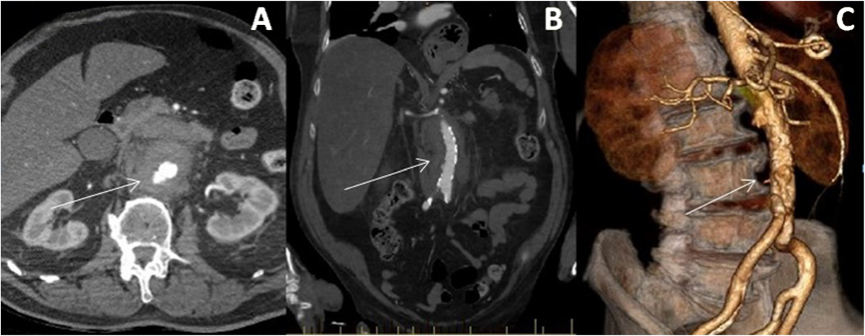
Imaging of the abdominal aorta prior to surgery. A. CT showing inflammatory tissue surrounding the leaking abdominal aorta (transverse plane). B. Coronal plane showing the abdominal aorta. C. Volume-rendered reconstruction of the abdominal aorta.
